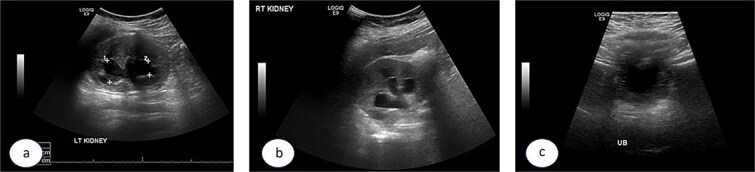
Post Deflux injection ultrasound images showing the left (a), and right (b) kidneys and the urinary bladder (c).
